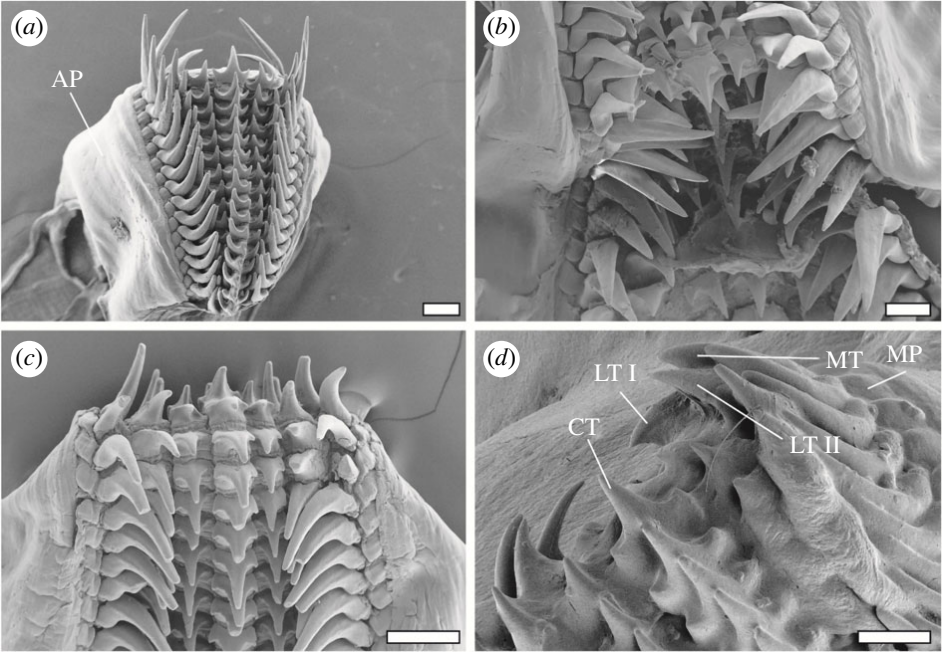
(*a–d*) Natural orientation of the teeth, when the alary processus is not ripped and the teeth are bent along the membrane by the alary processus (proposed feeding position). In (*b*,*d*), one can see that the marginal tooth can interact with the lateral II, potentially distributing stresses. The marginal plates seem to support the marginal teeth to the sides. Scale bars, 200 µm. AP, alary processus (= hyaline shield); CT, central tooth; LTI, lateral tooth I; LTII, lateral tooth II; MP, marginal plate; MT, marginal tooth.
